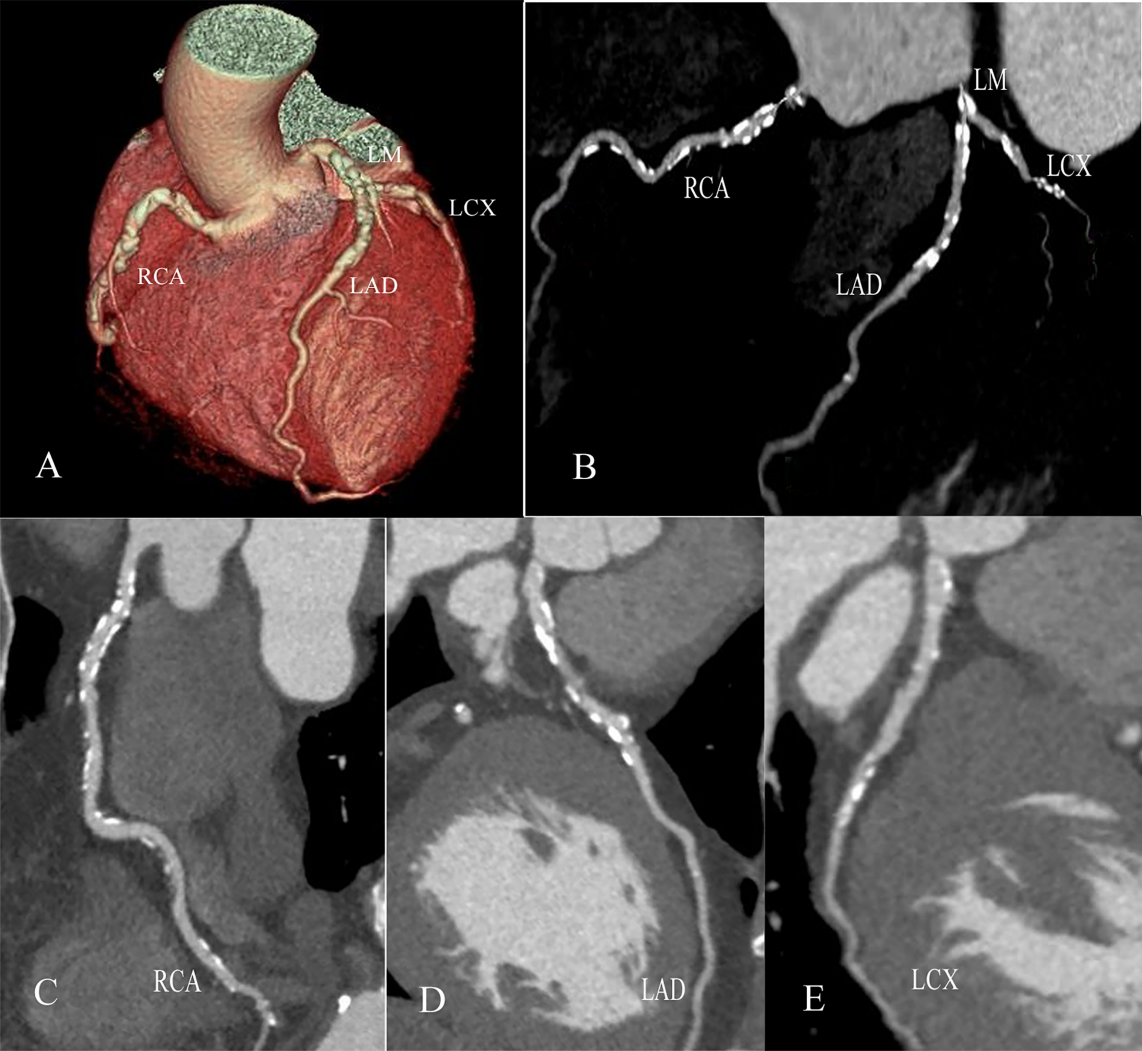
Multivessel disease in a male with type 2 diabetes mellitus with a 40-year smoking history. Volume rendering image (A), two-dimensional CT image (B) and curvature plane reconstruction images (C–E) show the nonsmooth edges and diffuse calcified and mixed plaques of the LM, LAD, RCA and LCX.
